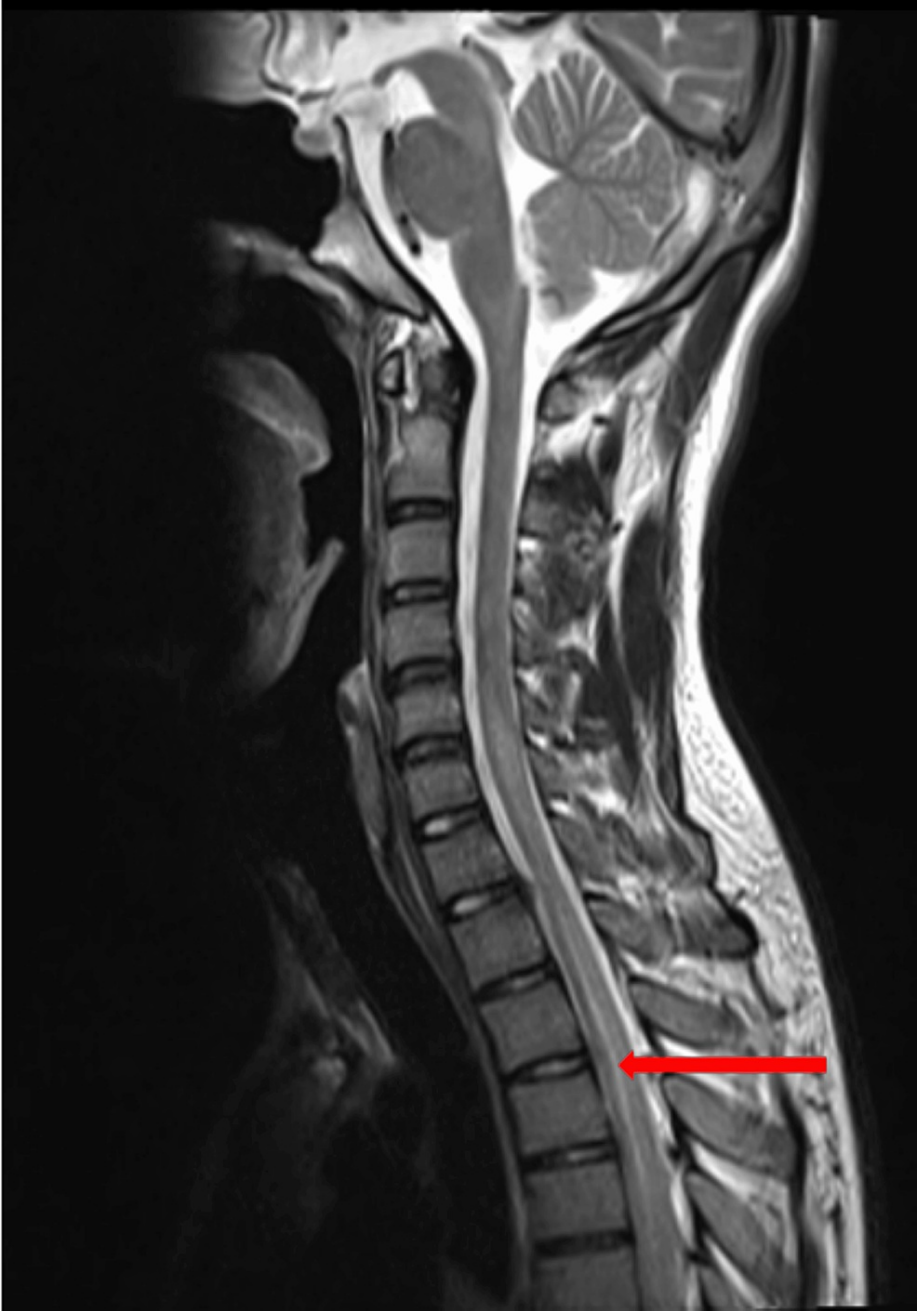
MRI whole spine with the dedicated cervical and lumbar region with contrast: T2/STIR long-segment ill-defined hyperintense signal noted in the cord extending from C2 vertebral body to upper half of L2 vertebral body with the possibility of transverse myelitis (red arrow).
